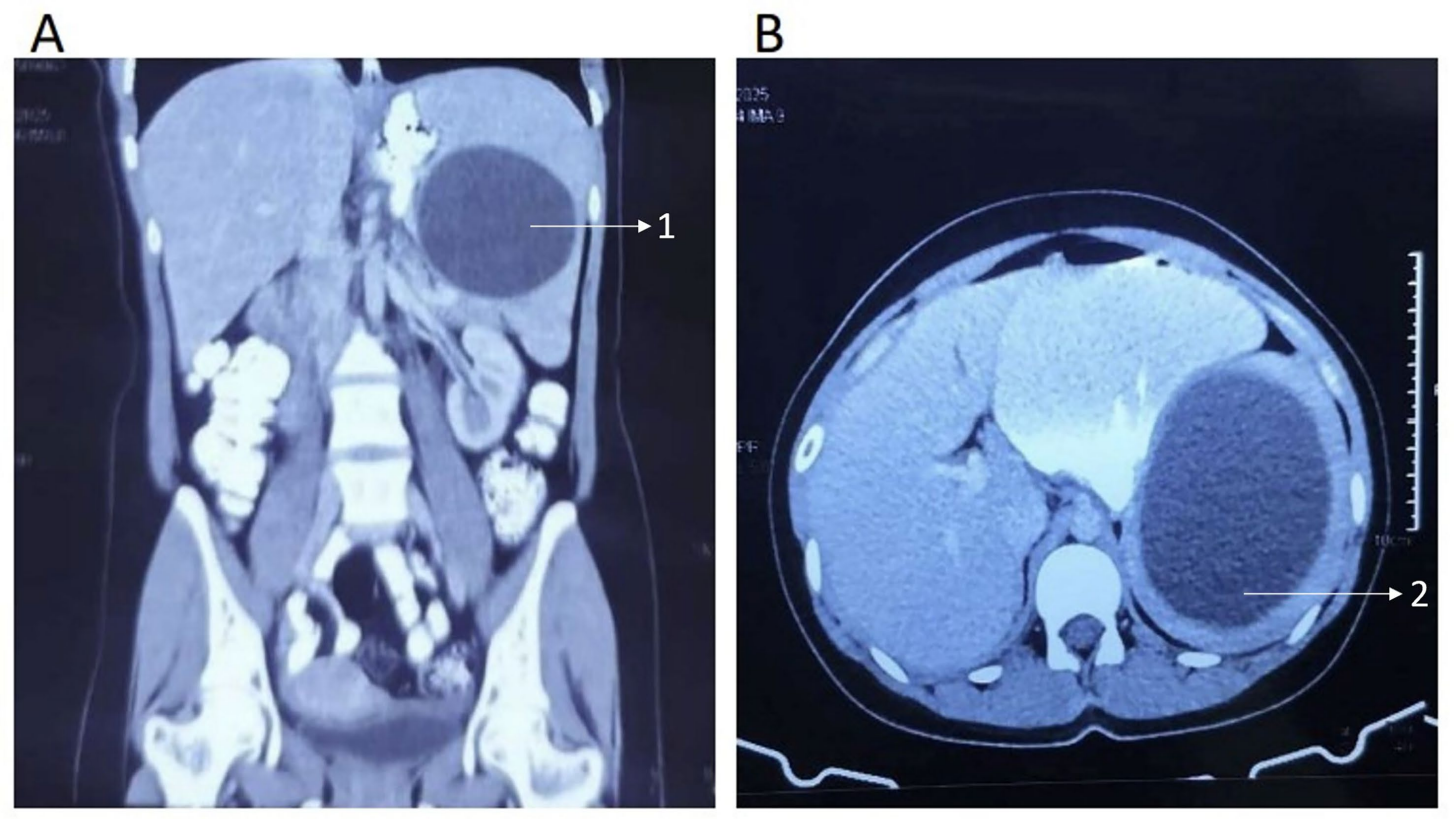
Contrast-enhanced computed tomography (CT) scan showing (A) coronal and (B) axial sections of the abdomen. A solitary cystic lesion is seen within the spleen, with no evidence of calcification or soft tissue component. (1) Solitary cyst with no internal septations on the coronal section. (2) Solitary cyst with no internal septations on the axial (transverse) section.
